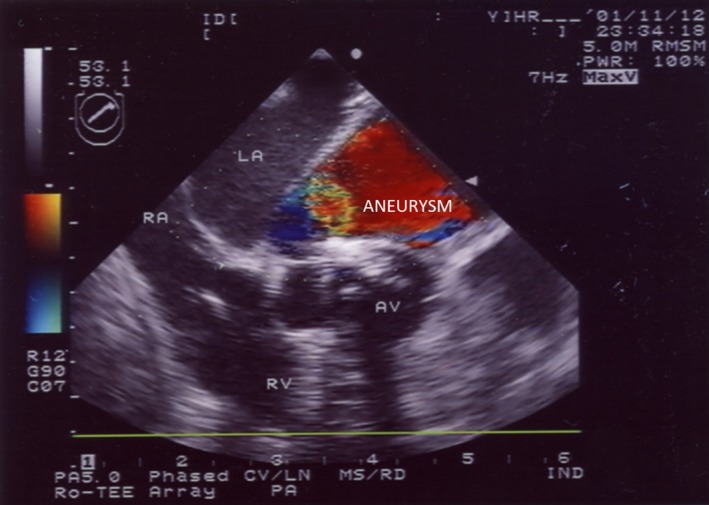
Transesophageal echocardiographic image (short‐axis view) of the aortic valve showing a left ventricular aneurysm lateral to the left atrium and calcified bicuspid aortic valve. AV, aortic valve; LA, left atrium; RA, right atrium; RV, right ventricle
